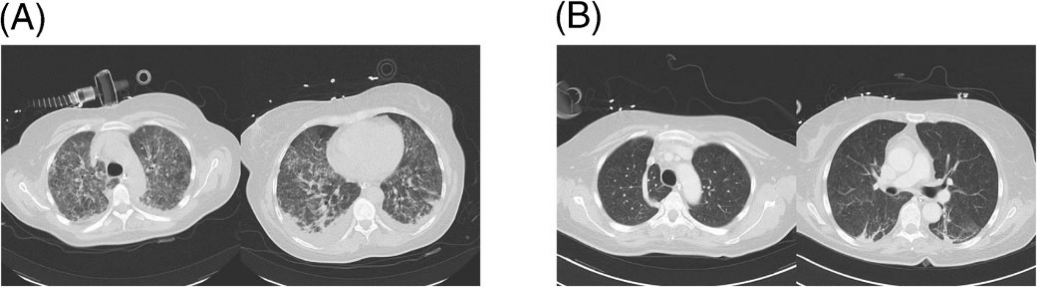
Initial CT scan (A) with bilateral diffuse interstitial nfiltrate and (B) the partial resolution after starting treatment.
